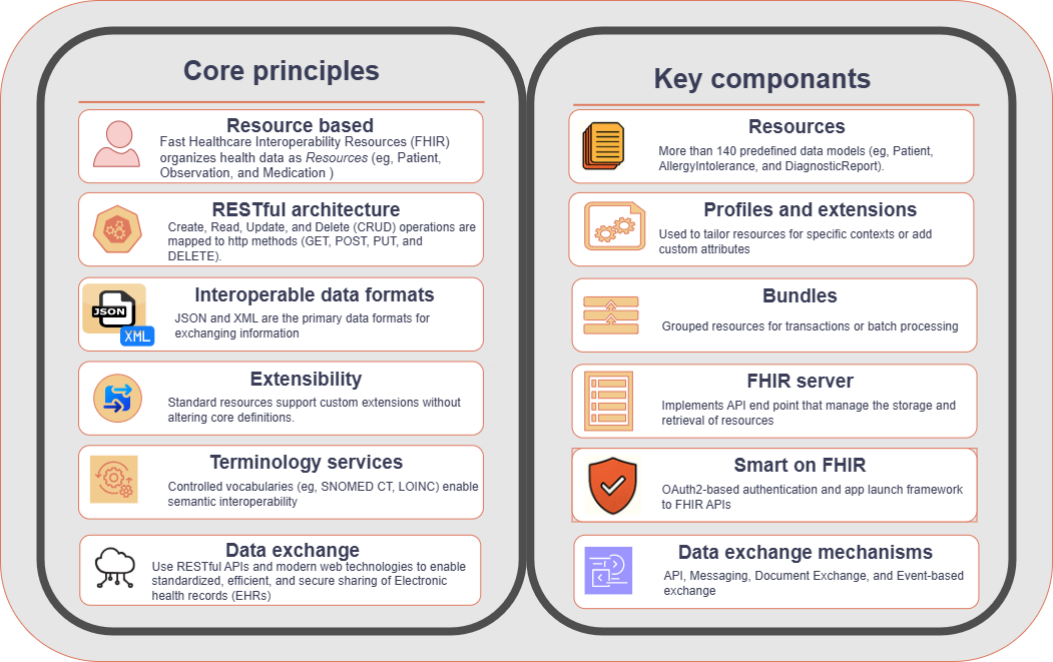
Fast Healthcare Interoperability Resources (FHIR) technical overview. API: application programming interface; CRUD: basic operations performed on the data: create, read, update, and delete; EHR: electronic health record; LOINC: Logical Observation Identifiers Names and Codes; OAuth2: open authorization protocol version 2.0; SNOMED CT: Systematized Nomenclature of Medicine–Clinical Terms.
